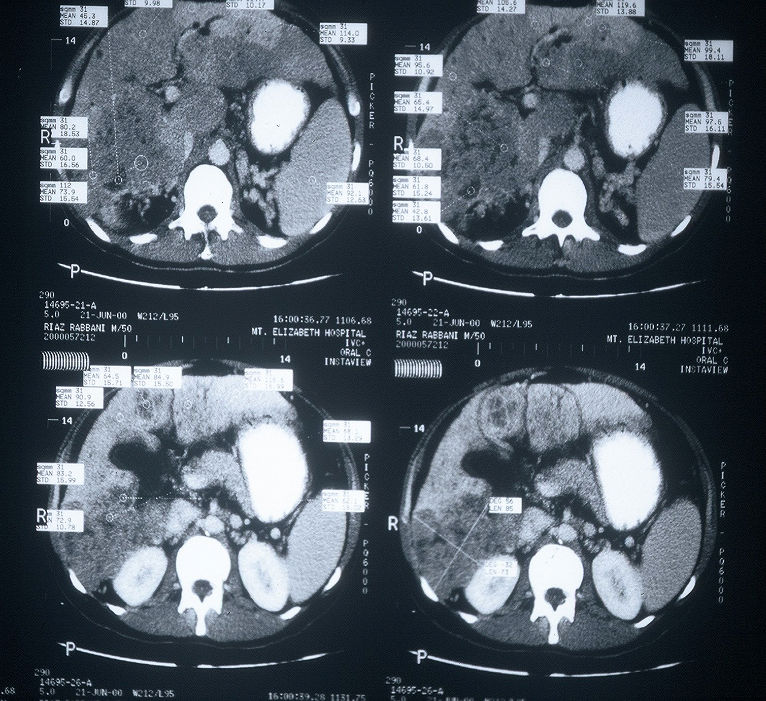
CT scan slices of multifocal liver tumour.
